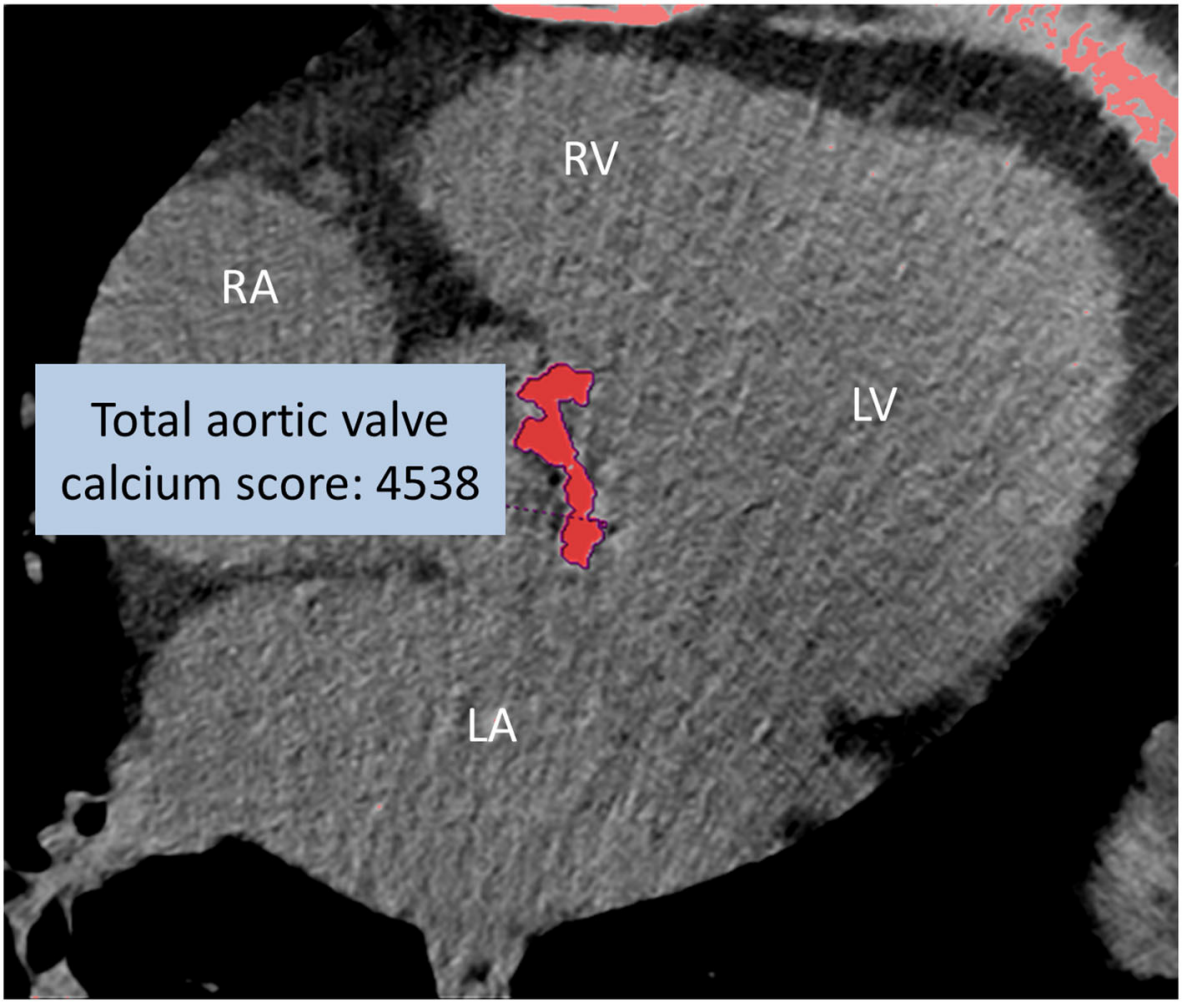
Non-enhanced CT of severe aortic valve calcification (total AVCS: 4538). Calcium scoring of the aortic valve using post-processing software by the Agatston method. RA, right atrium; RV, right ventricle; LA, left atrium; LV, left ventricle.
